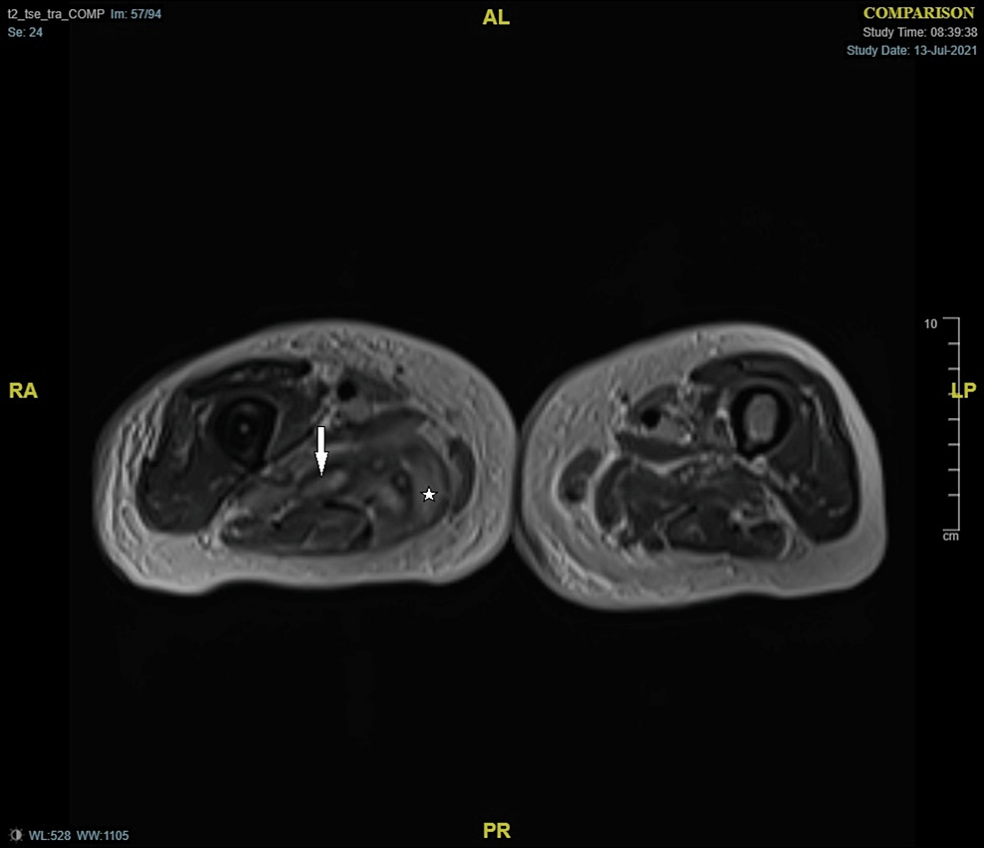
MRI thigh - T2-weighted image Arrow: Muscle collection; Star: Tissue oedema
